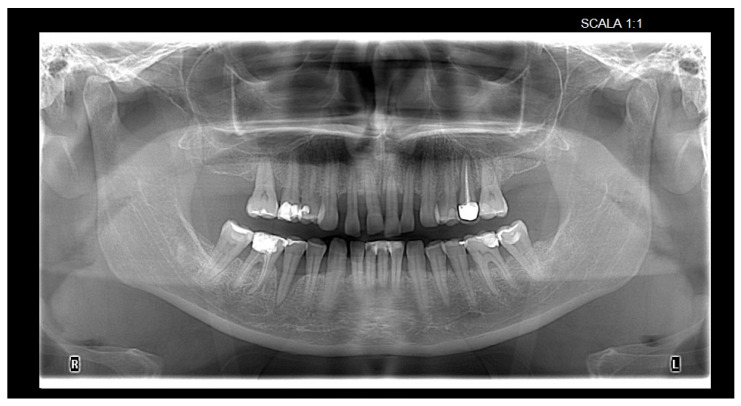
Orthopantomogram evaluation.
